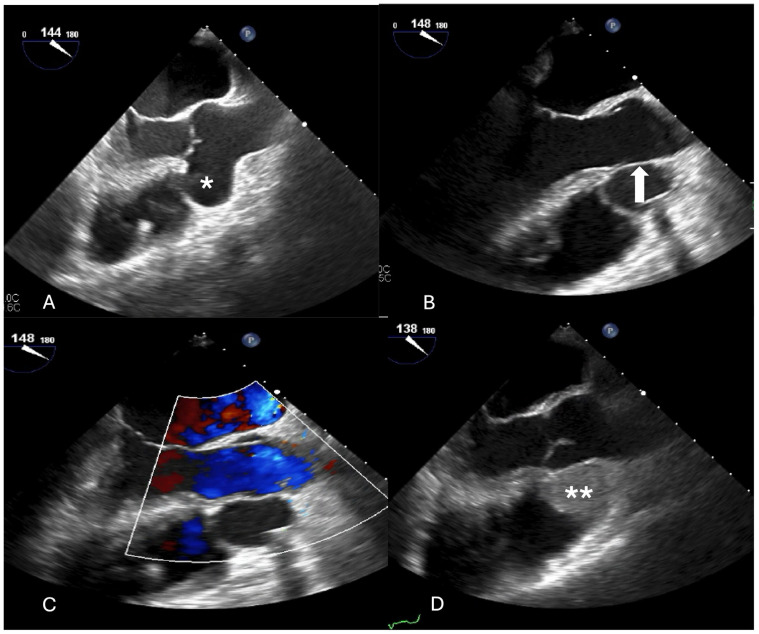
(A) Large aneurysm of the right sinus of valsalva with compression of the left ventricular outflow tract (white *). (B) Closure with a large patch (white arrow) to exclude the aneurysm (resection not possible). (C) Intraoperative transesophageal echocardiography showing normal flow condition in the left ventricular outflow and aortic root without turbulences into the former aneurysm cavity. (D) Before closing the chest, the aneurysm cavity had already thrombosed (white ).
